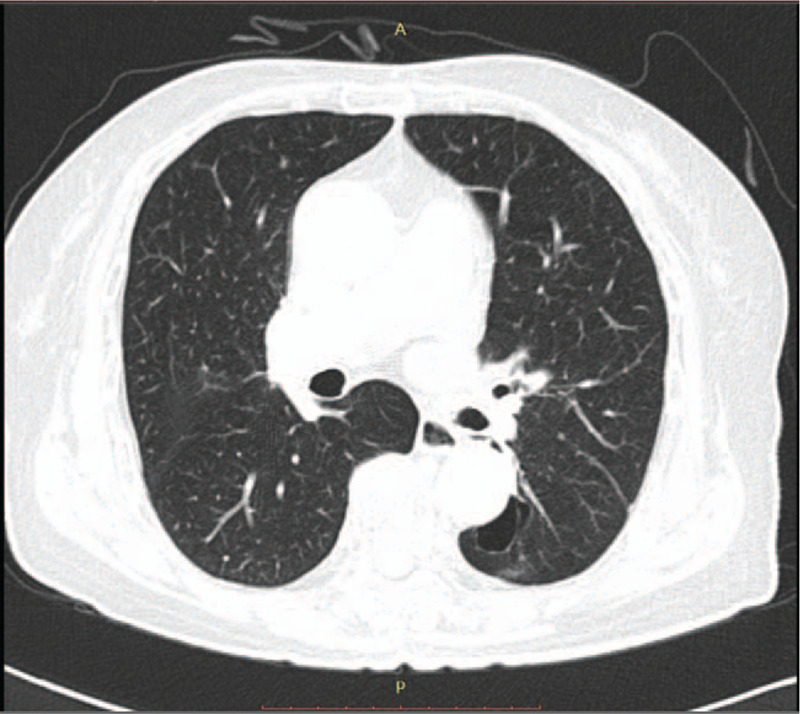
A recent chest computed tomography scan on June 8, 2020, indicated that the partial response was sustained at 78 months on the combined gefitinib and herbal medicine treatment.
